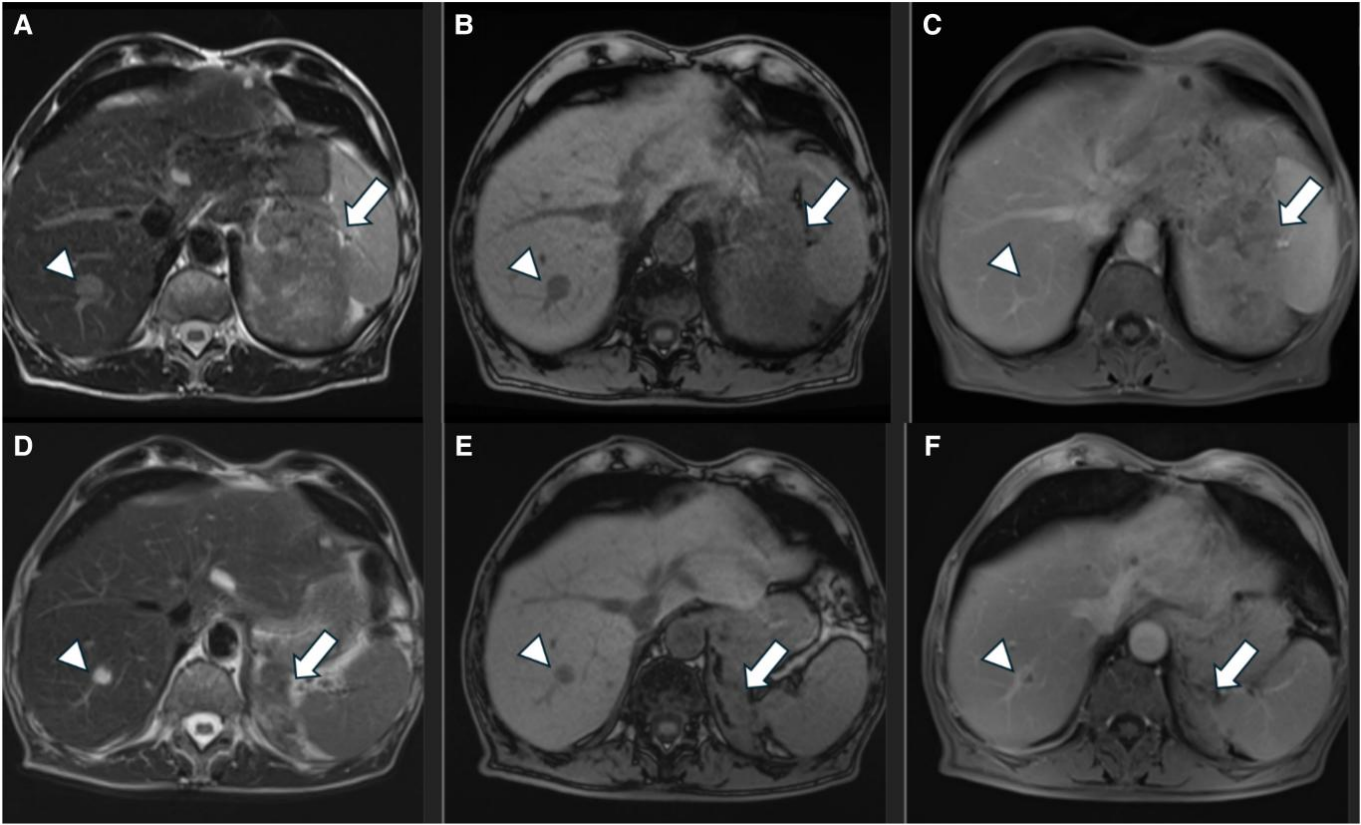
Pre-and 6 months posttreatment MRI of the same patient. Axial T2W STIR (A), T1-opposed phase (B), and contrast-enhanced T1W SPIR (C) images on pretreatment MRI demonstrate a heterogeneous left adrenal mass (arrow in A and B) with intense contrast enhancement (arrow in C). A hepatic metastasis is also seen in the right liver lobe (arrowheads in A-C). Corresponding posttreatment MRI exhibits a significant decrease in the adrenal lesion size (arrows in D-F). Note the minimal enhancement of the lesion on the postcontrast image (arrow in F), another finding indicative of the tumor's response to treatment. Liver lesion is also decreased (arrowheads in D and E) and less enhanced (arrowhead in F) on posttreatment MRI. Abbreviations: MRI, magnetic resonance imaging.
